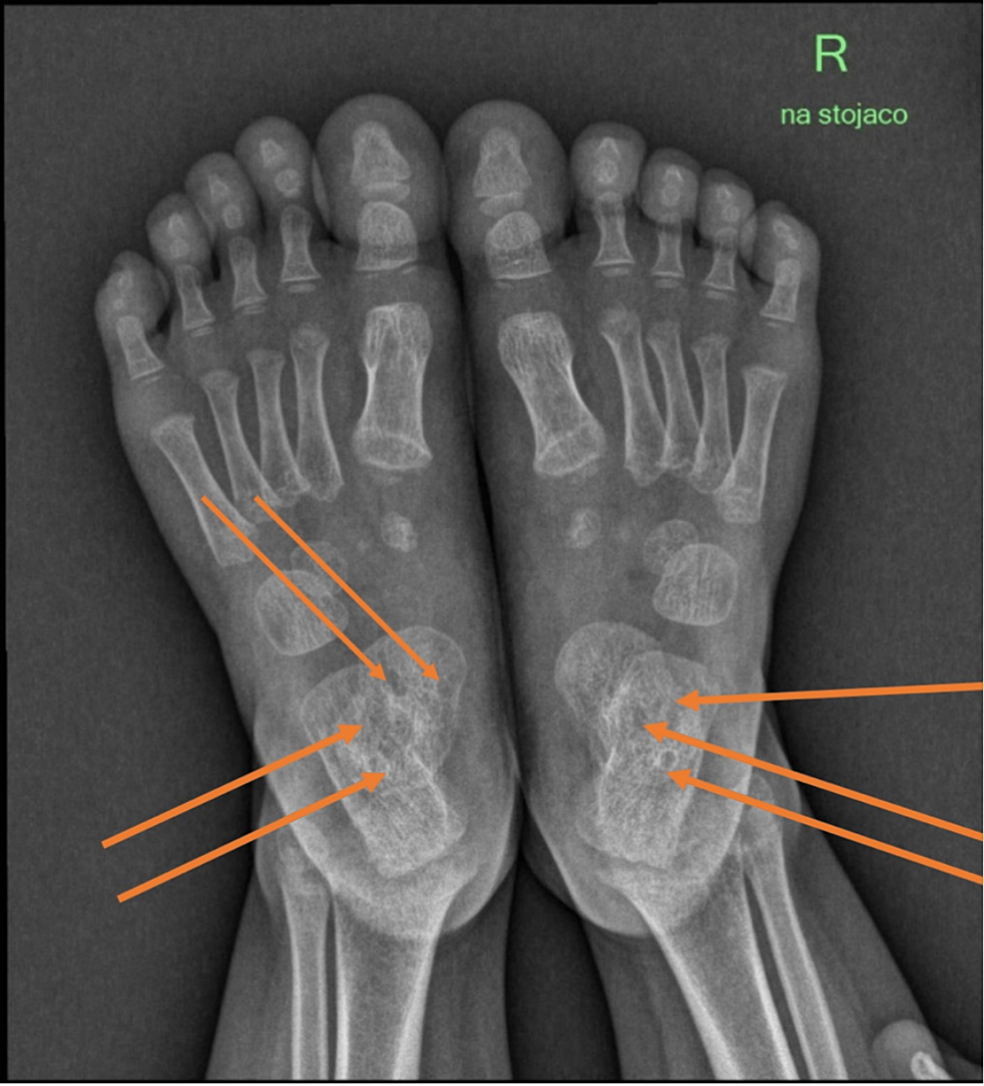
Anterior-posterior (AP) X-rays of the feet before treatment The arrows highlight the poor bone density areas with bone cysts and cavitations
